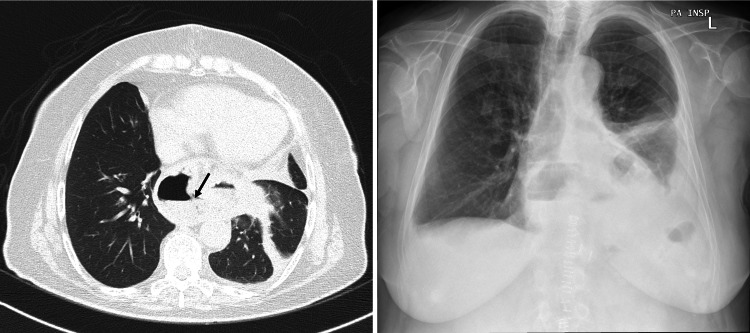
Re-evaluation with thoracic CT scan and chest X-ray showing a small sliding esophageal hiatal hernia (black arrow)
